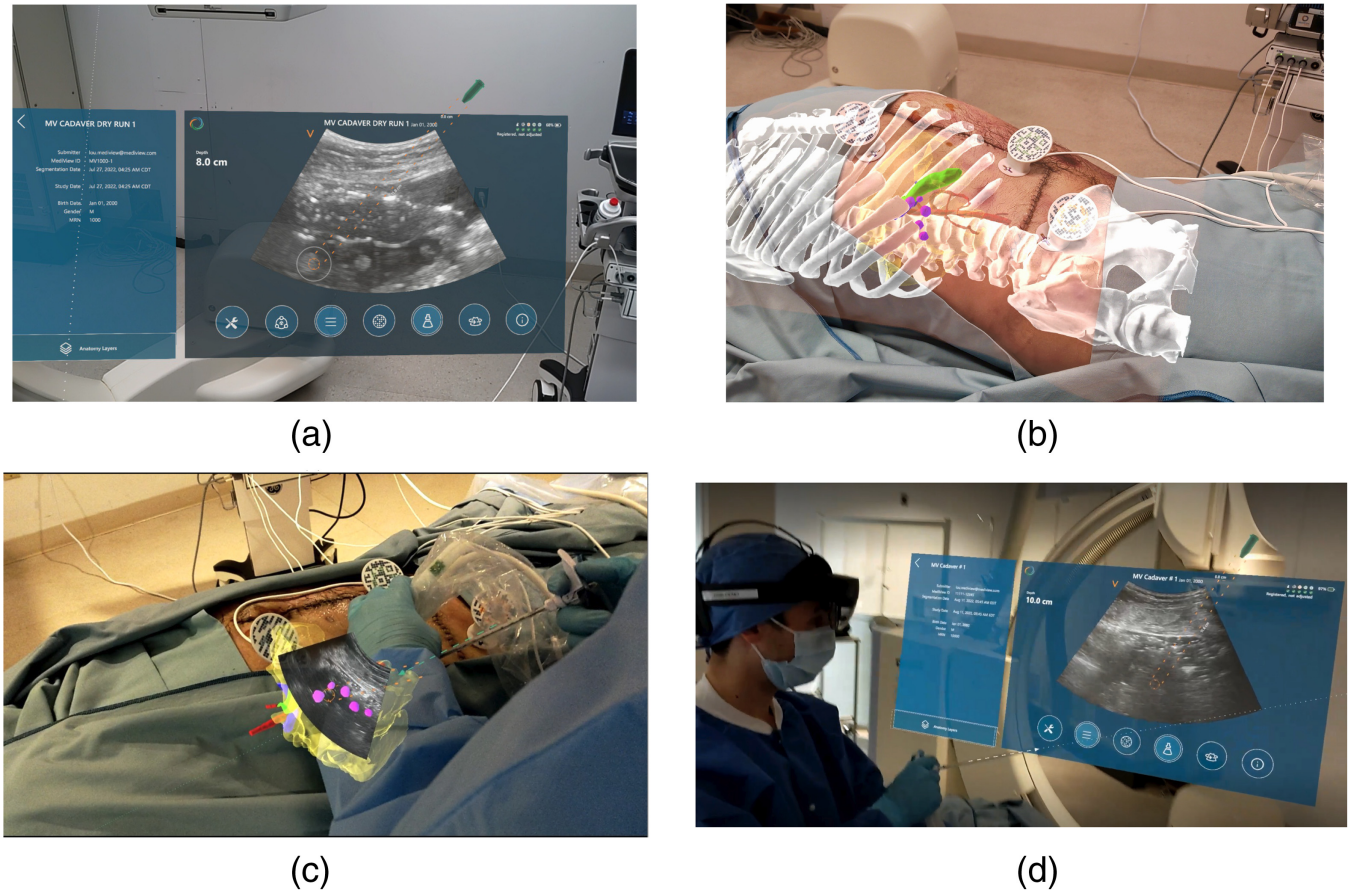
First-person views of primary system projections. (a) HUD featuring streamed US and needle guide directly from US. HUD may be positioned based on operator preference allowing for ergonomically friendly positioning. (b) Stereoscopic 3D CT anatomy registered to the subject and registered US projection. Five purple circular projections are the stereoscopic projection of implanted targets. (c) Registered US projection intersecting with stereoscopic CT targets, virtual needle trajectory (green), and EM-tracked needle with needle guide (orange). Three circular registration markers are also shown encircling the region of interest. (d) First-person view of a second operator using the HUD display to define a needle trajectory during the study. The image was taken by a user also wearing a headset so that the HUD was visible.
